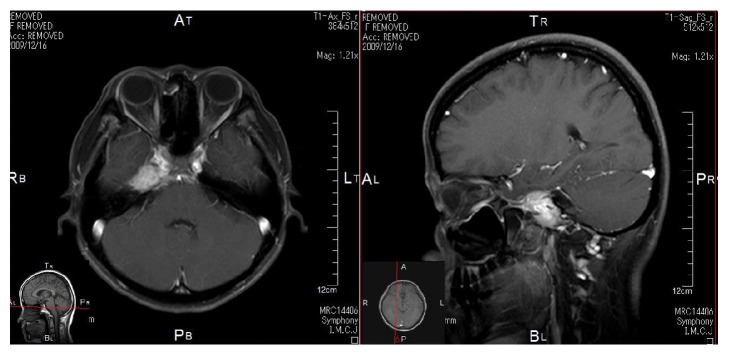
MRI finding of the patient with multiple myeloma who had abducens nerve palsy. MRI images show soft tissue mass enhanced with Ga in the region of the apex of the pyramid.
